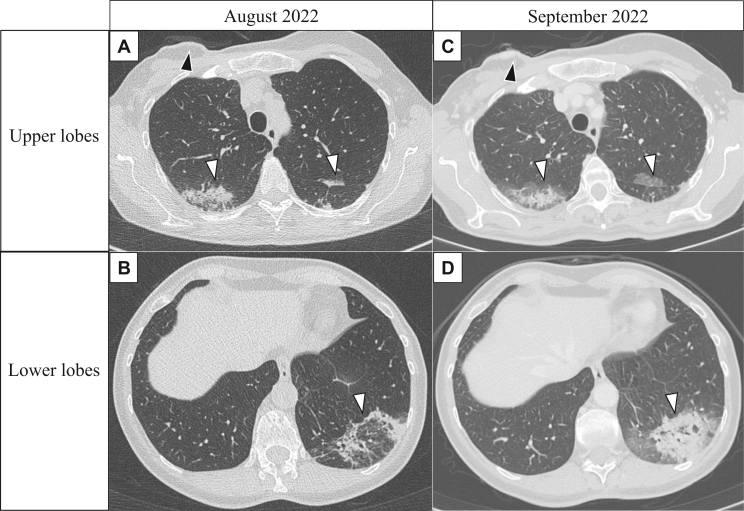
Example of interstitial lung disease induced by trastuzumab deruxtecan therapy in a 53-year-old female patient with T4 breast cancer at the Parma Hospital. The images show patterns of interstitial lung disease evidenced in a non-contrast computed tomography (CT) scan carried out in August 2022 (A and B), radiologically worsened in a subsequent contrast enhanced CT scan carried out 1 month later in September 2022 (C and D). A cryptogenic organizing pneumonia (COP) pattern characterized by ground-glass opacities and lung consolidations (white arrowheads) can be observed in the most dependent portions of both the upper lobes (A and C) and in the posterior basal segment of the left lower lobe. In the upper lobes (A and B) it is also possible to observe the dimensional growth of the breast cancer located in the right breast, associated with skin involvement.
